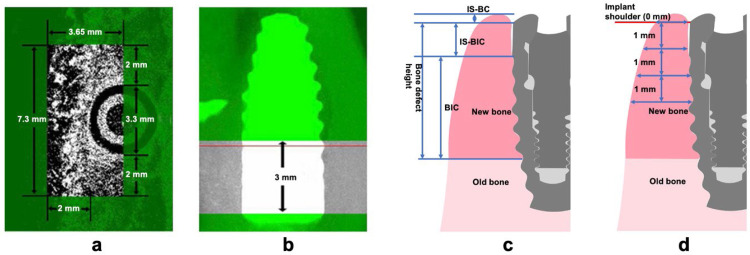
Measurement methods used in radiographic analysis and histomorphometric analysis. A and B, In the radiographic analysis, the VOI dimensions on the buccal side of the implant were 7.3 mm by 3.65 mm. C and D, The linear measurements for the histomorphometric analysis are shown in the vertical and horizontal directions. VOI, volume of interest.
